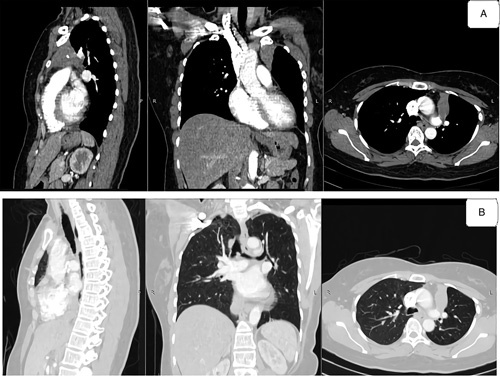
Computed tomography scan thorax contrast.
